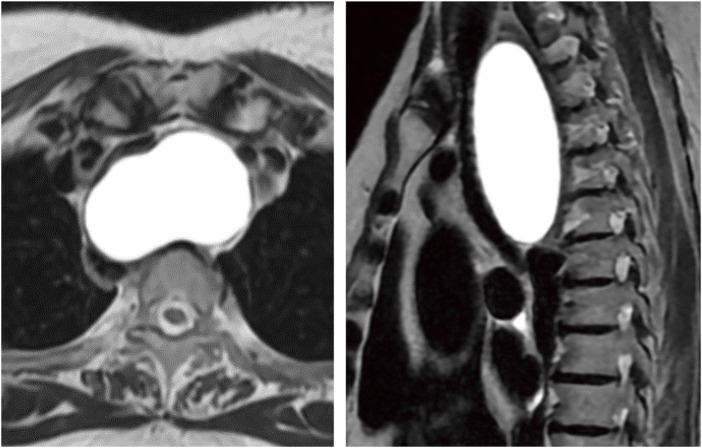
MRI showing a homogeneously hyperintense signal mass in the superior mediastinum on the T2-weighted images.
